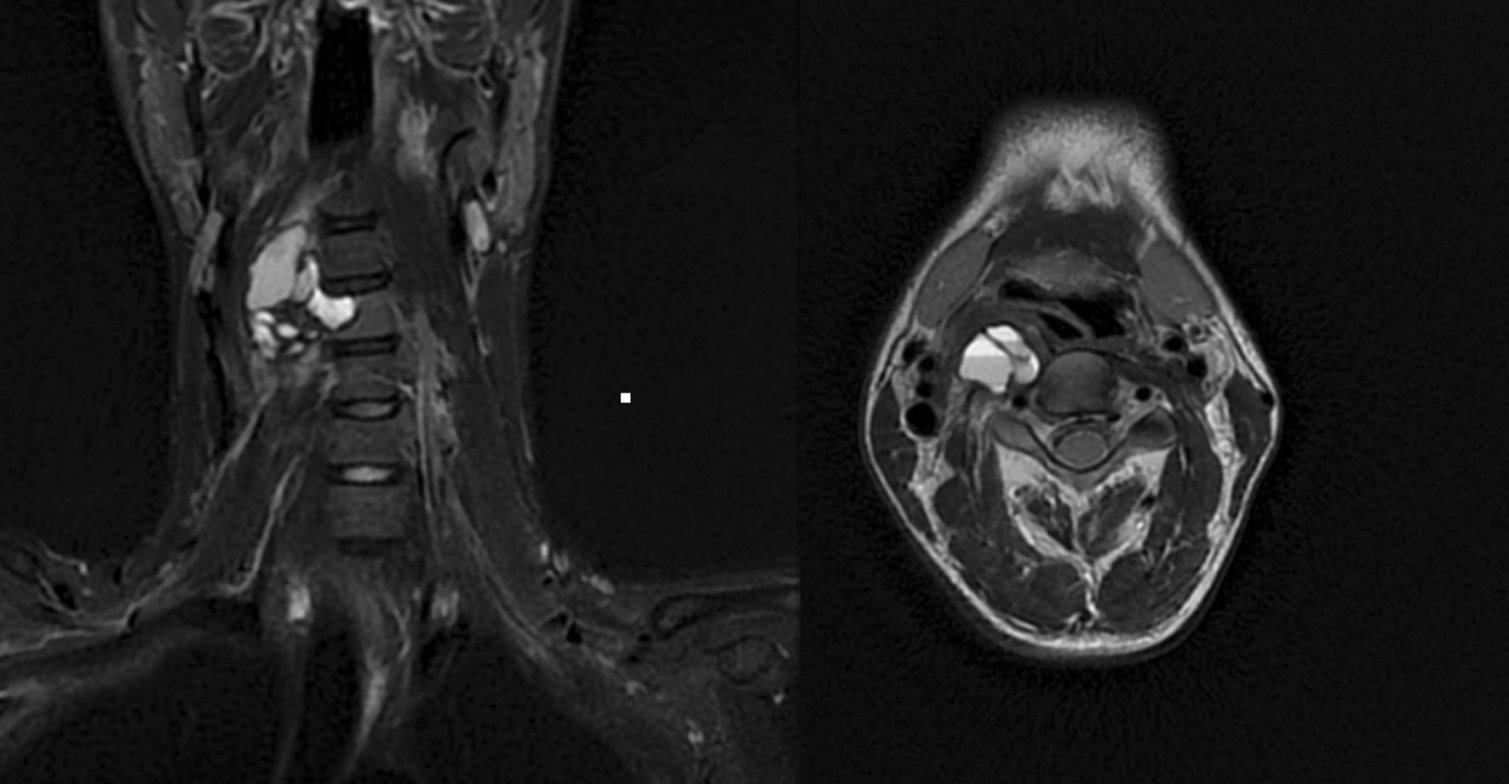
Pre-interventional MRI depicting an aneurysmal bone cyst of the fourth cervical vertebra, around 2,2 × 3,5 × 4,2 cm, with characteristic blood-fluid levels
